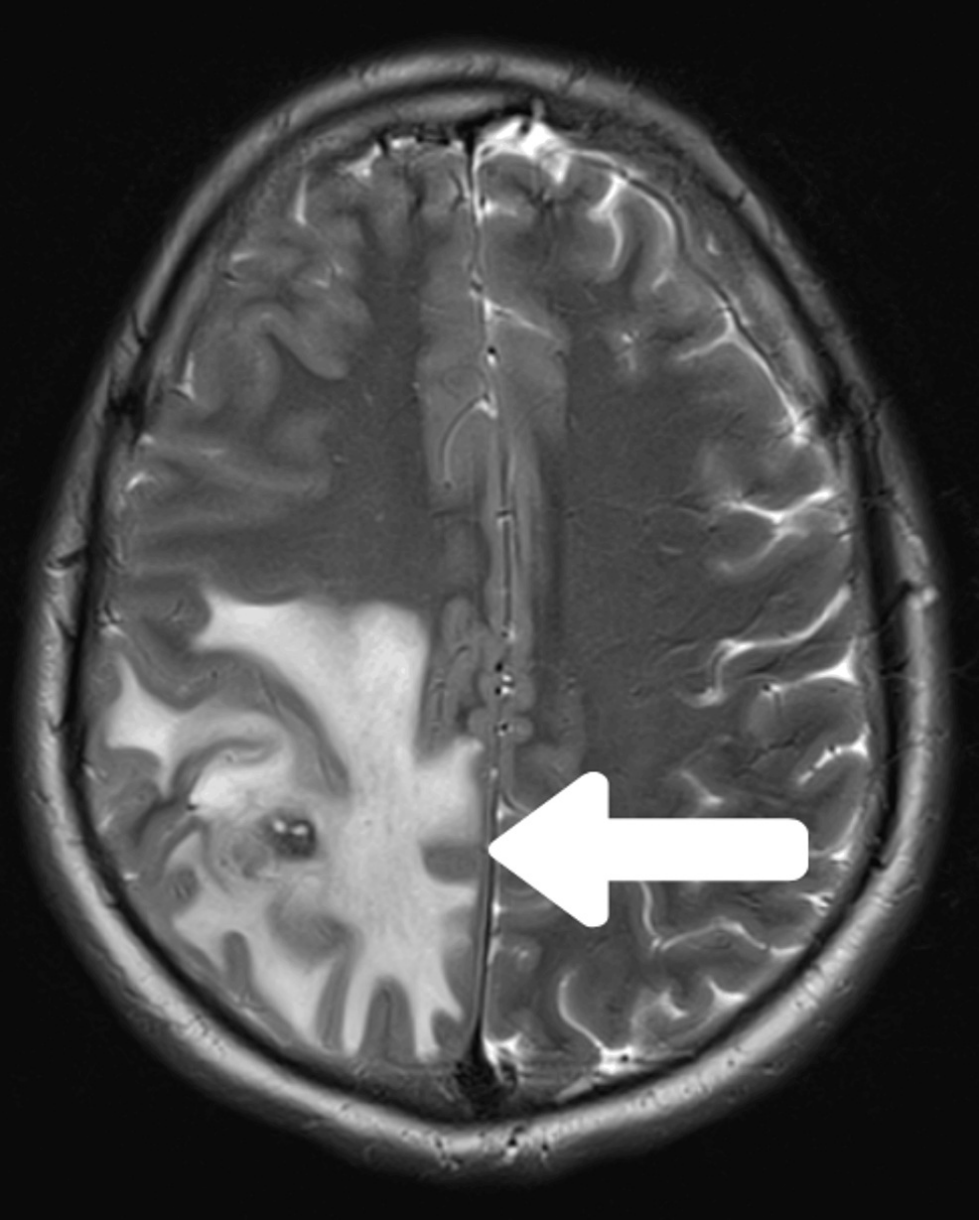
T2-weighted MRI sequence T2-weighted MRI sequence showing hyperintense core with perilesional edema (white arrow). MRI: magnetic resonance imaging
